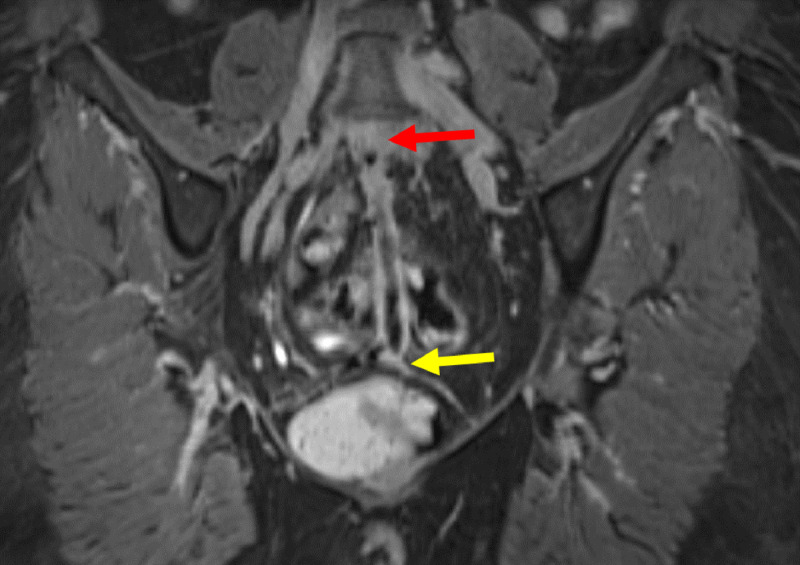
Coronal 3D contrast MRI in T1 fat saturation weighing: Full path of fistula from L5-S1 (yellow arrow) to the vagina (red arrow). The fistula presents with a hyperintense wall surrounding a hypointense lumen.
